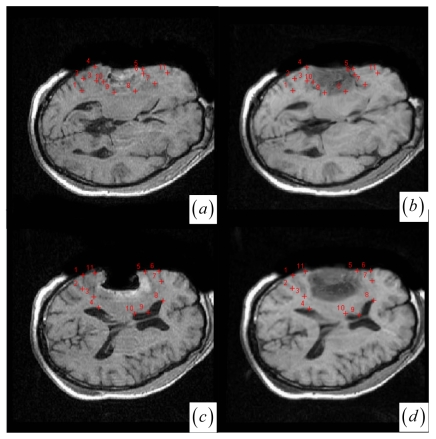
(a)–(b) Corresponding landmarks for intra- and pre-operative MR images at Figure 3(a)–(b). (c)–(d) Corresponding landmarks for intra- and pre-operative MR images at Figure 4(a)–(b).
